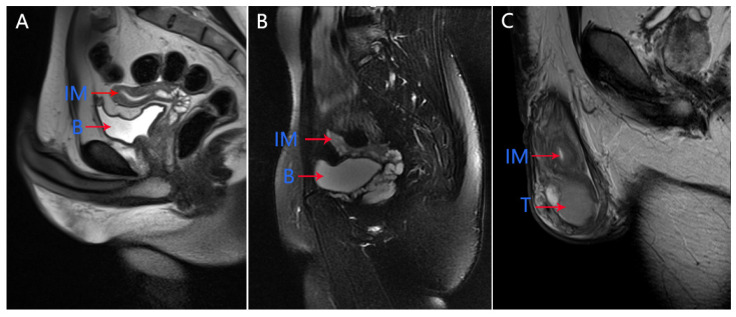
Pelvic magnetic resonance images of the three cases. Pelvic magnetic resonance images show Müllerian remnants in cases 1 (A), 2 (B), and 3 (C). IM, immature uterus; B, bladder; T, testis.
